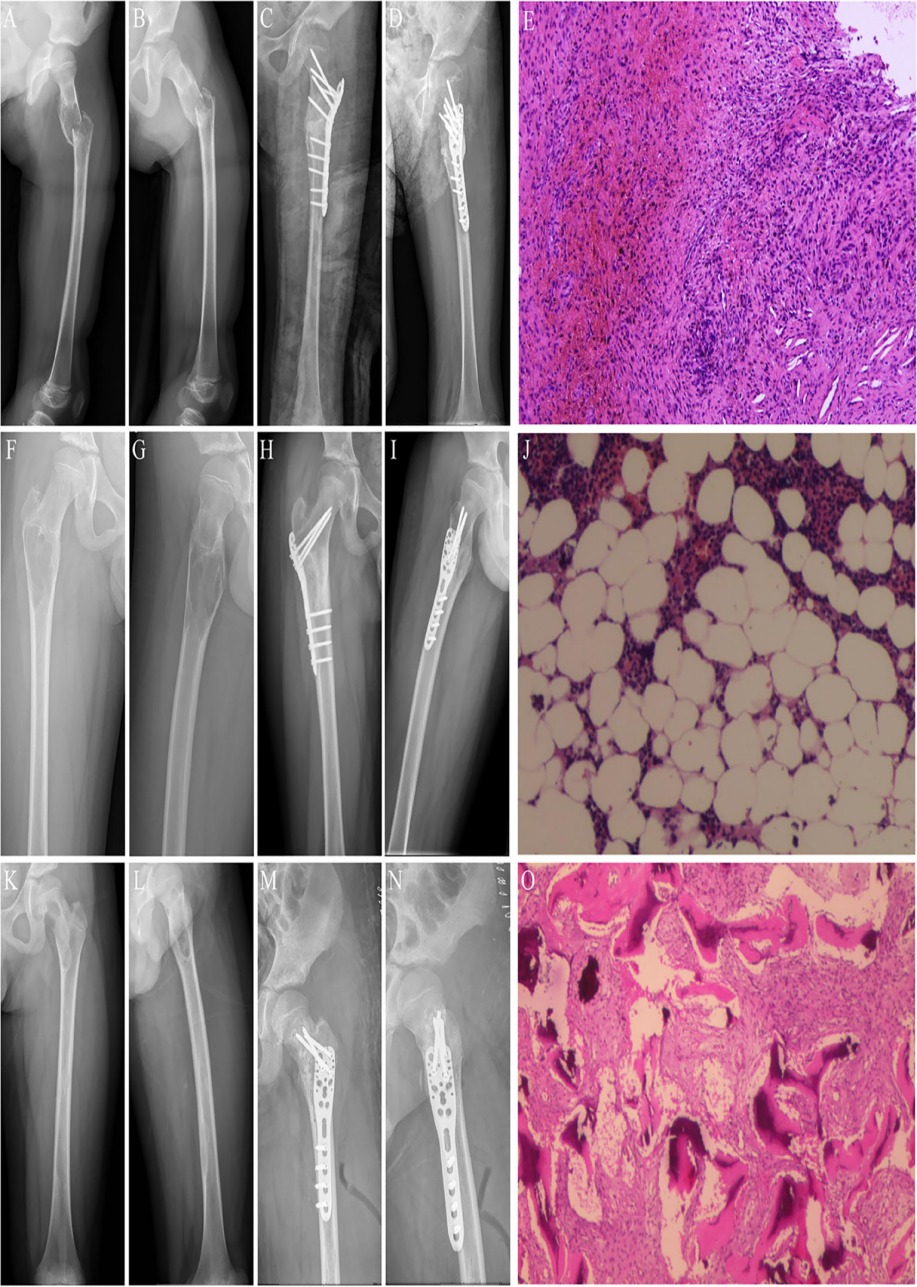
The three types of benign lesions were treated in this study. (A) Preoperative posteroanterior x-ray image of a patient with a pathological fracture of the left proximal femur caused by BC. (B) Preoperative lateral x-ray image of a patient with a pathological fracture of the left proximal femur caused by BC. (C) Postoperative posteroanterior x-ray image of a patient with BC of the left proximal femur. (D) Postoperative lateral x-ray image of a patient with BC of the left proximal femur. (E) Postoperative pathologic image of BC. (F) Preoperative posteroanterior x-ray image of a patient with a pathologic fracture of the left proximal femur caused by ABC. (G) Preoperative lateral x-ray image of a patient with a pathological fracture of the left proximal femur caused by ABC. (H) Postoperative posteroanterior X-ray image of one patient with ABC of the left proximal femur. (I) Postoperative lateral x-ray image of a patient with ABC of the left proximal femur. (J) Postoperative pathological image of ABC. (K) Preoperative posteroanterior x-ray image of one patient with a pathological fracture of the left proximal femur caused by FD. (L) Preoperative lateral x-ray image of a patient with a pathological fracture of the left proximal femur caused by FD. (M) Postoperative x-ray image of a patient with FD of the left proximal femur. (N) Postoperative lateral x-ray image of a patient with FD of the left proximal femur. (O) Postoperative pathologic image of FD.
